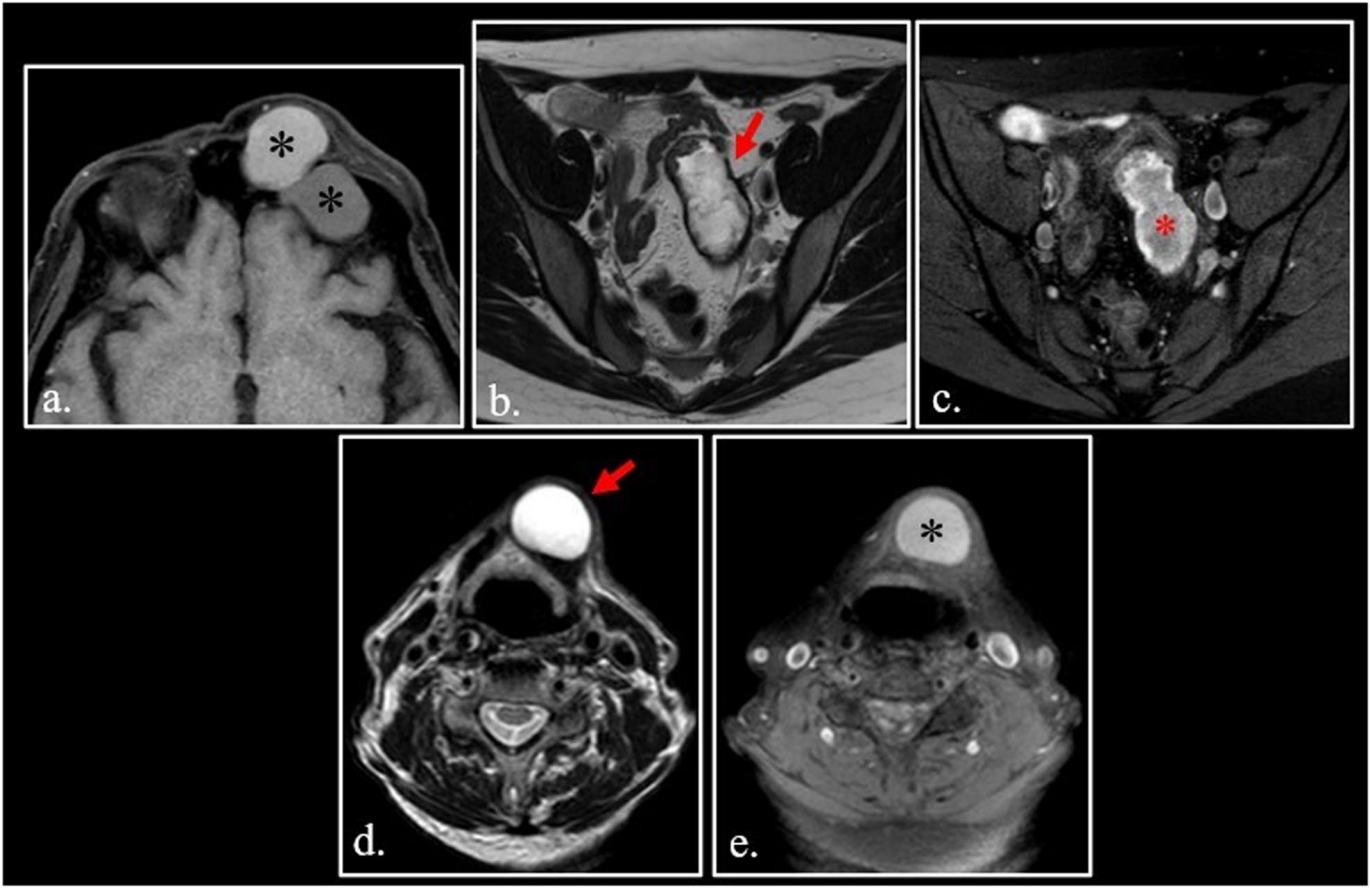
a FSE T1-weighted fat-sat scan shows two mucoceles of the left frontal sinus. Different concentration of glycoproteins explains the different hyperintensity of the two lesions (asterisks). b, c FSE T2-weighted scan (b) shows a cystic mucinous borderline cistoadenoma of the left ovary (arrow) with disomogeneous hyperintensity (asterisk) on T1 weighted fat-sat scan (c). d, e FSE T2-weighted (d) and T1-weighted fat-sat (e) scans of a thyreoglossal cyst (arrow) abutting right strap muscles. T1 hyperintensity (asterisks) correlates to the high content of thyreoglobulin
